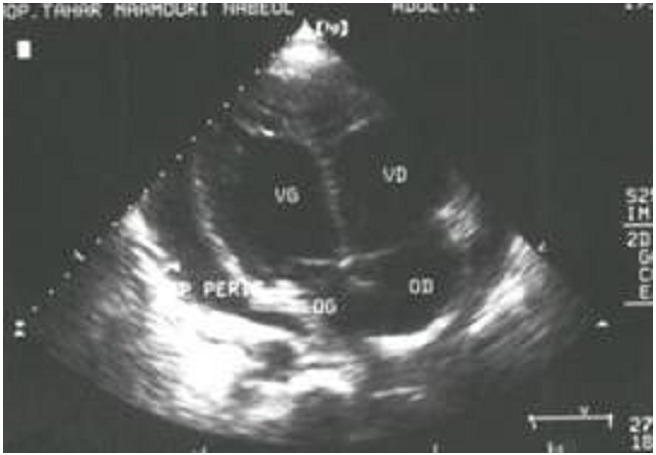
Echocardiography practiced in emergency confirming the hemopericardium
